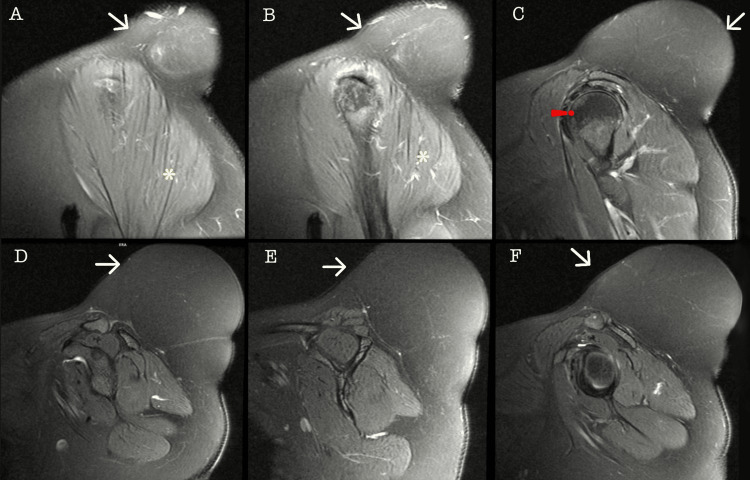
Magnetic resonance imaging in coronal section in T2 The white arrow indicates a hypointense tumor, which is compared to muscle and isointense to fat. The red line delineates the humeral head, while the yellow asterisk denotes the deltoid muscle.
